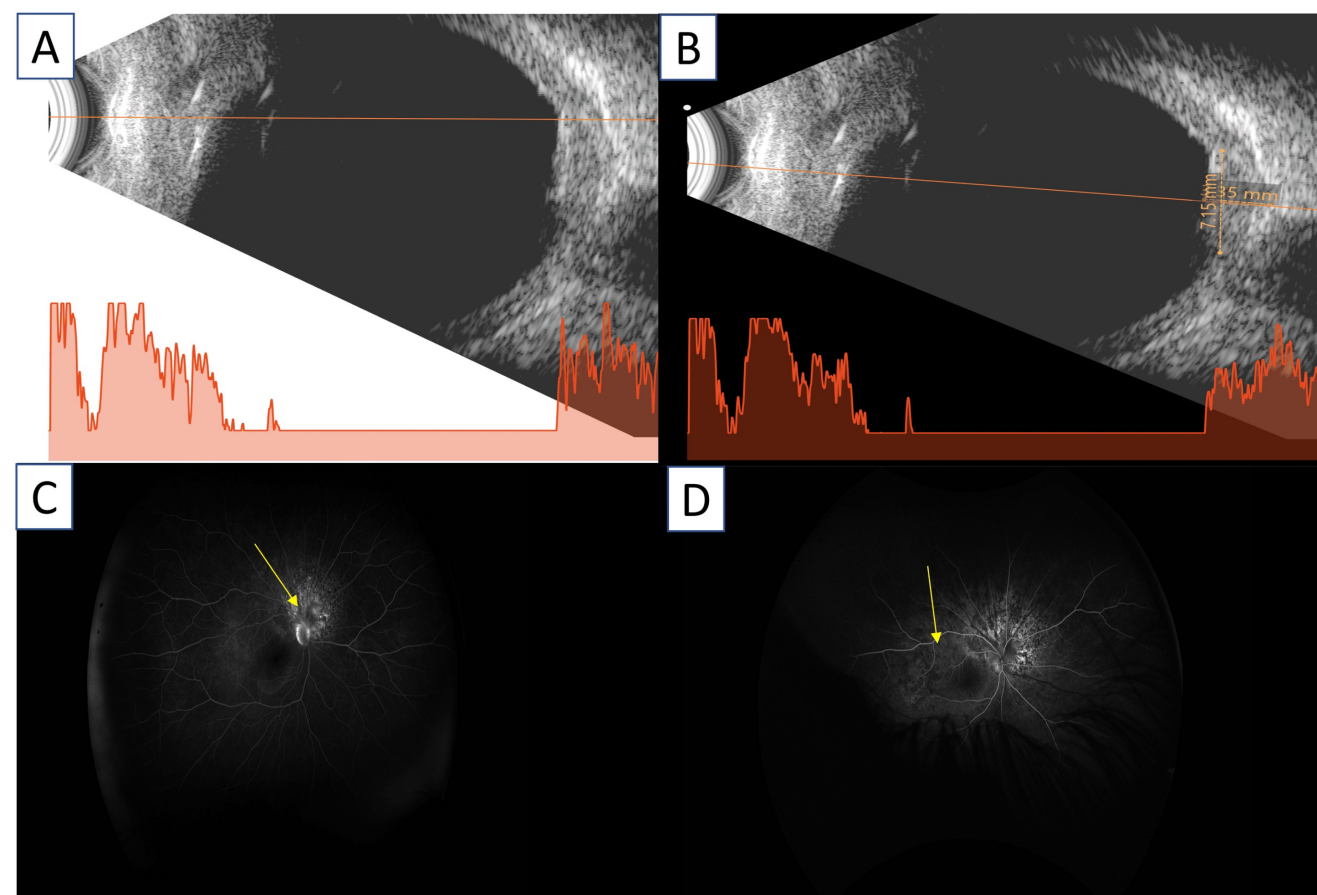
A & B: B-scan ultrasonography demonstrated an acoustically solid, homogenous intraocular lesion measuring 6.4 mm at its base and 2.35 mm in thickness. C & D: Fundus fluorescein angiogram depicting early hyperfluorescence of the lesion with increasing intensity in the mid-phase and late leakage of dye (yellow arrow).
